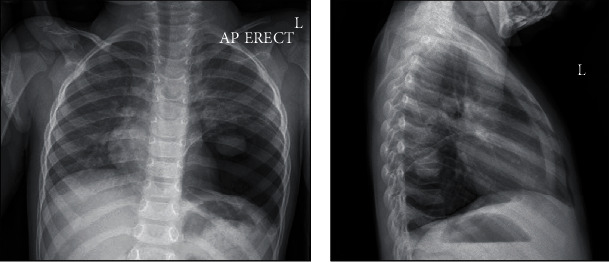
Anteroposterior and lateral chest X-ray showing cystic lesion at left lower zone.
